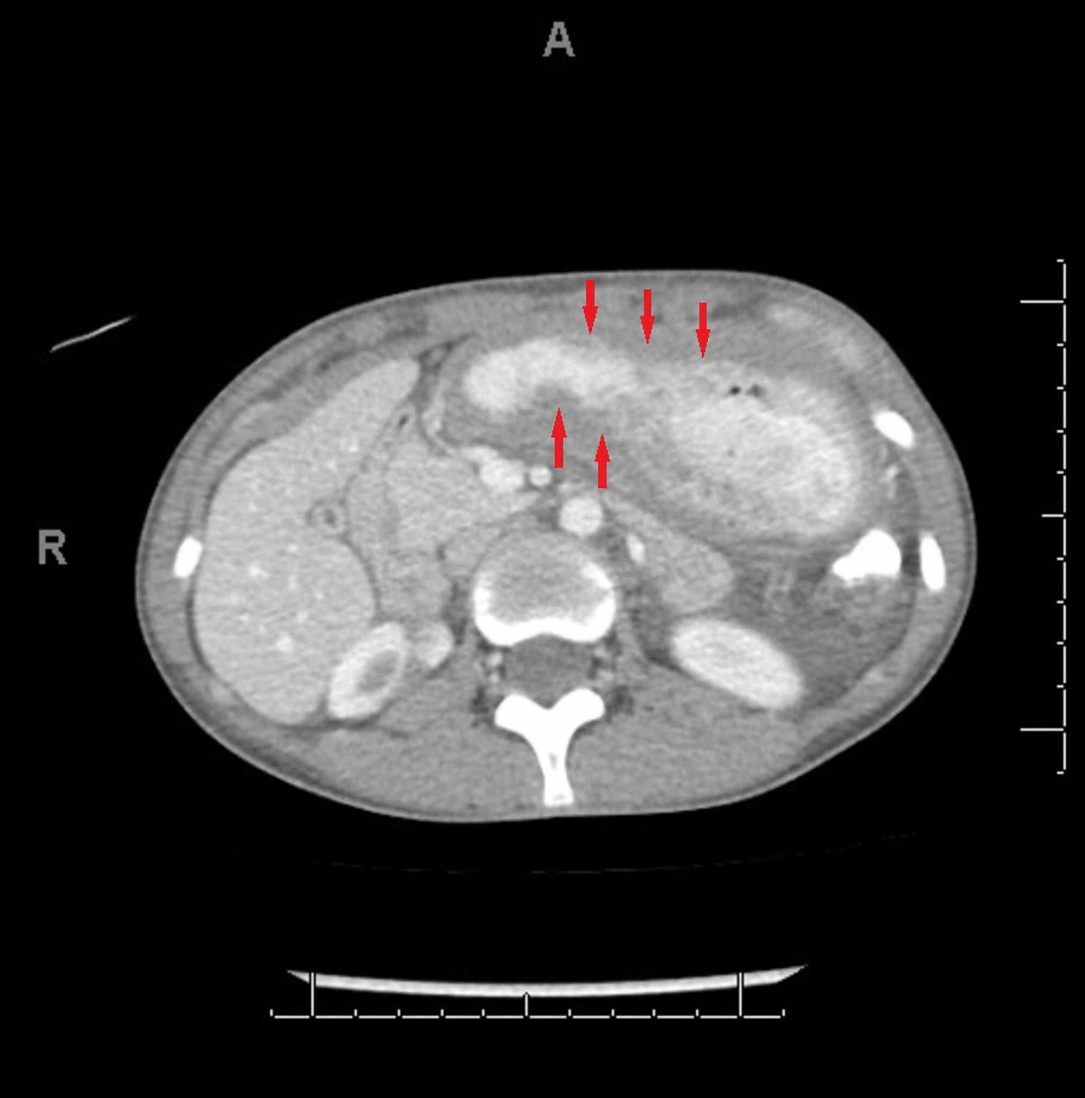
CT scan axial view: arrows indicate extensive infiltration of the stomach wall with the malignant process
